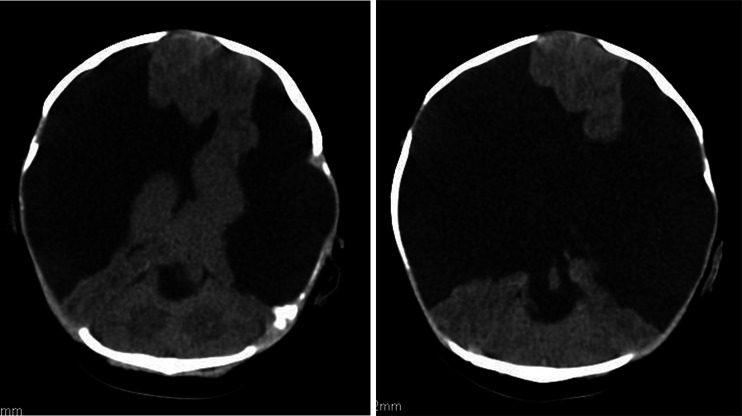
Bilateral open-type schizencephaly. The compressed sulci and longitudinal fissure of the brain represent signs of intracranial hypertension. Nonenhanced CT scan
